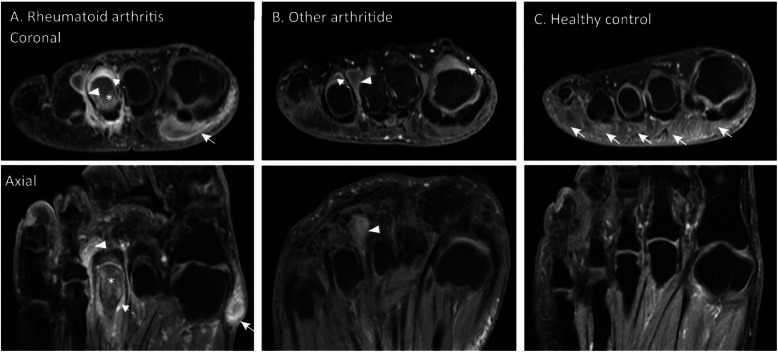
MR examples of intermetatarsal and submetatarsal bursae. Coronal and axial fat suppressed T1-weighted FSE gadolinium-enhanced images of the forefoot at the level of the metatarsal heads. a Female participant with RA (age 61 years) with intermetatarsal (IMB) and submetatarsal bursitis (SMB). IMB in the 3rd intermetatarsal space (arrowhead) with peripheral enhancement protruding dorsal (dumbbell shape) and plantar (teardrop shape) of the metatarsal heads. Peripheral enhancement of a mass in the first submetatarsal space, consistent with SMB (white arrow). Synovitis of MTP 3 (dotted arrow), as well as osteitis in the head of the third metacarpal bone and proximal phalanx (*). b Female participant with another arthritide (diagnosis of viral reactive arthritis, age 34 years) and IMB at the 3rd intermetatarsal space (arrowhead) with dorsal protrusion. Additional synovitis of MTP 1 and 4 (dotted arrows). c Female healthy control (age 50 years) with diffuse submetatarsal alterations (DSMA) in all submetatarsal spaces, predominantly visible at the 1st, 2nd, and 4th submetatarsal spaces (arrows). Intense linear contrast enhancement at the 2nd intermetatarsal space is consistent with a small vessel on the consecutive slices (not shown), there is no IMB visible on the axial images
